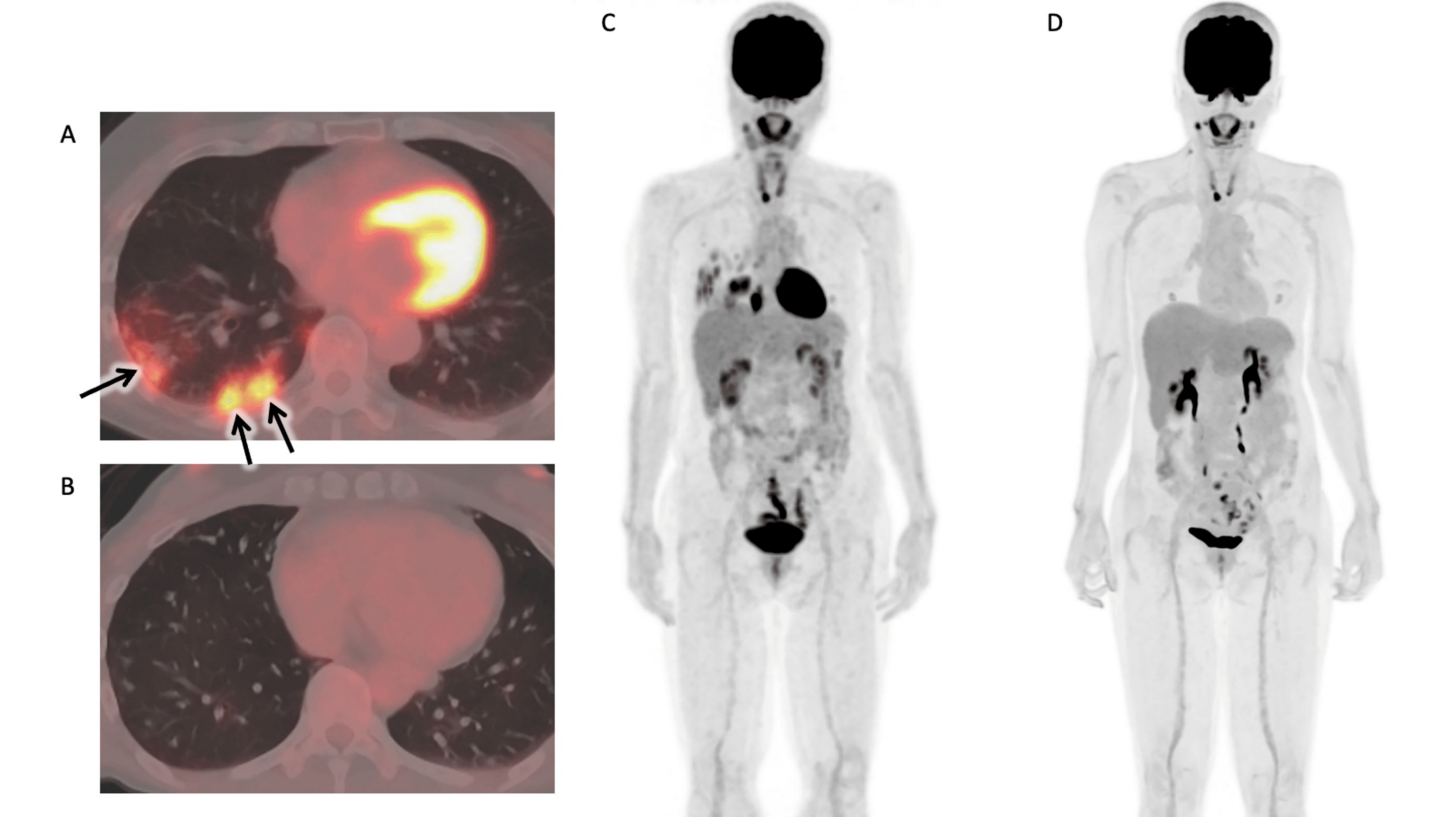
FDG-PET/CT images of a representative case (Case 8) who responded to anti-mycobacterial therapy. Prior to treatment initiation, high FDG uptake is observed in multiple nodules (black arrows) within the right lower lobe (A: axial image; C: coronal image). At 52 weeks post-treatment, FDG uptake exhibited a near-complete reduction (B: axial image; D: coronal image). FDG-PET/CT: fluorodeoxyglucose positron emission tomography/computed tomography
